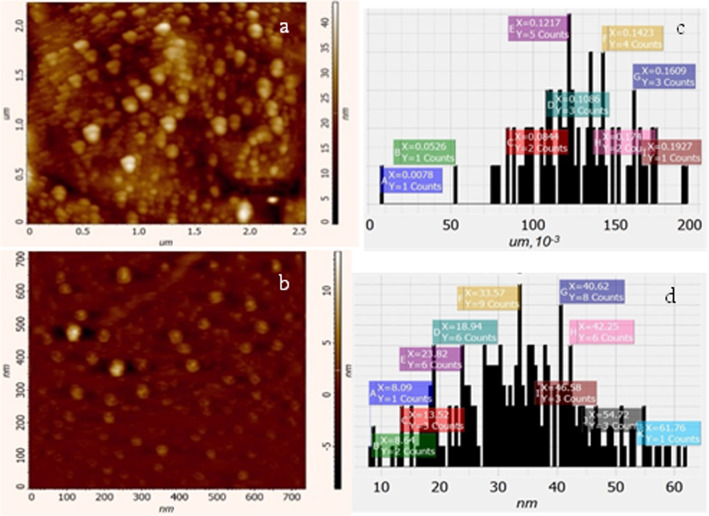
AFM images of non-treated (ultrasound-treated a (b)) and average size distribution c (d) of Ag NPs
